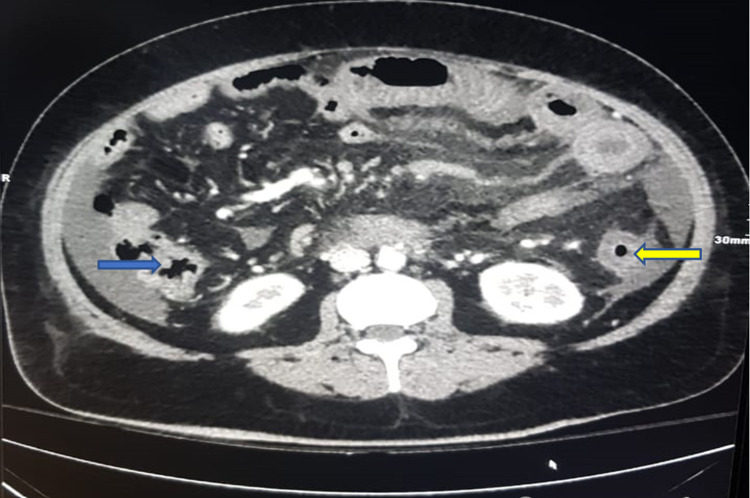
Computed tomography showing mural thickening at the hepatic flexure (blue arrow) and splenic flexure (yellow arrow).
